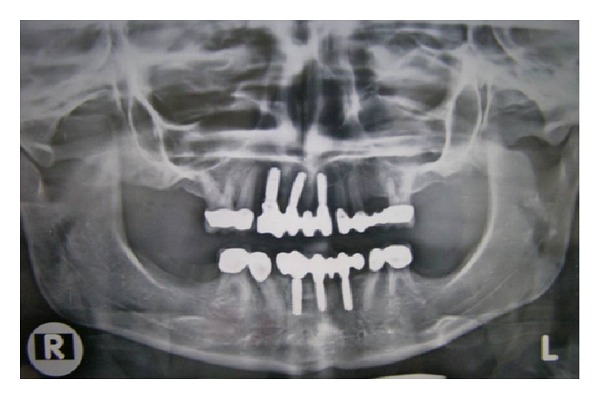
Radiograph taken after 6 months of cementation of the prosthesis.
